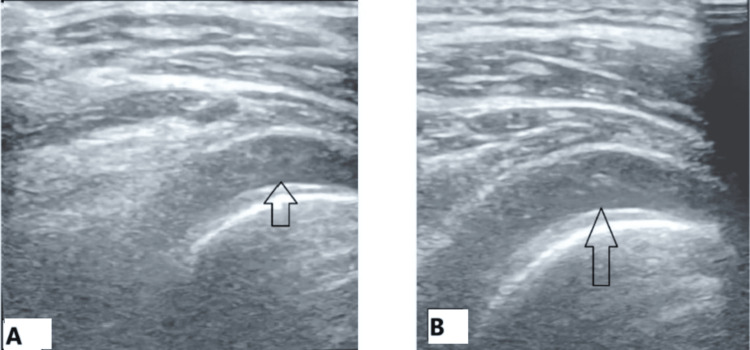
(A) Hydrodilatation of the right shoulder joint. (B) Joint space in ultrasound before dilatation. A significant increase in the joint space after hydrodilatation is shown
